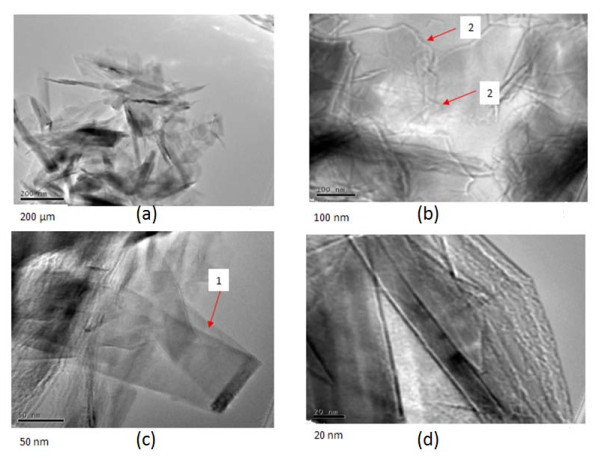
TEM images. TEM images without ultrasonic oscillation (a) large FLG edges, (b) and (d) folded FLG, (c) large graphene sheet with rolled edge.
